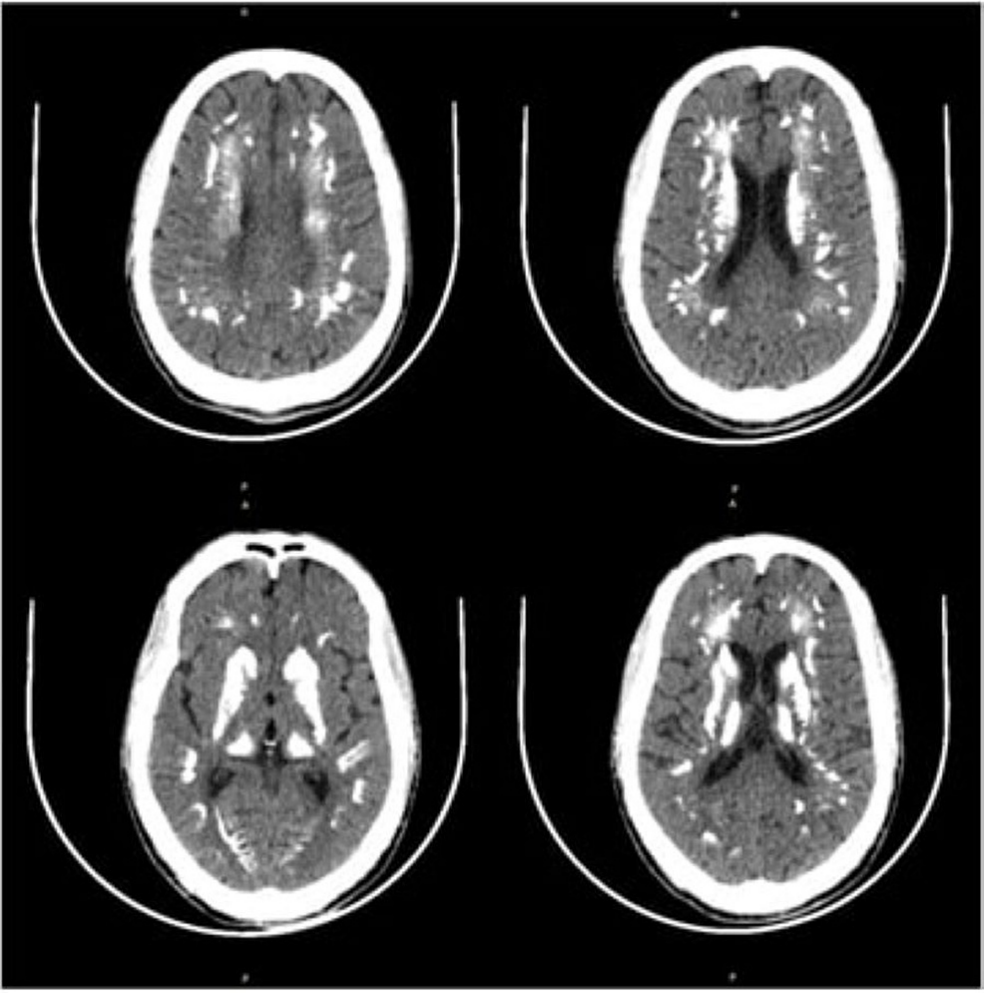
Non-contrast CT of the brain (axial CT images) Bilateral symmetrical, dense, and extensive calcification in subcortical white matter, periventricular gray matter, centrum semiovale, caudate nucleus, basal ganglia, internal capsule and thalamus.
